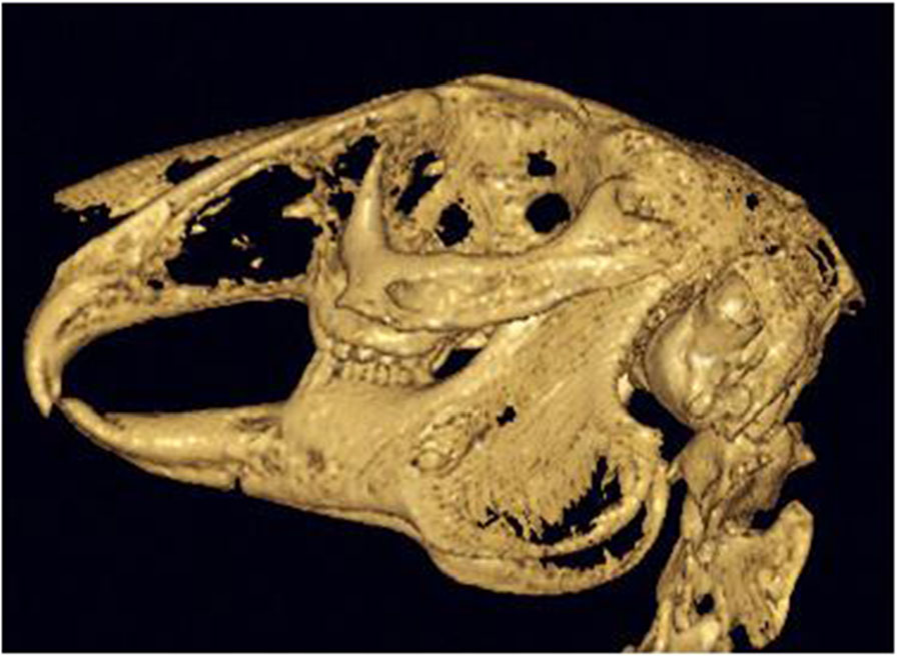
The result of CBCT examination after surgery
